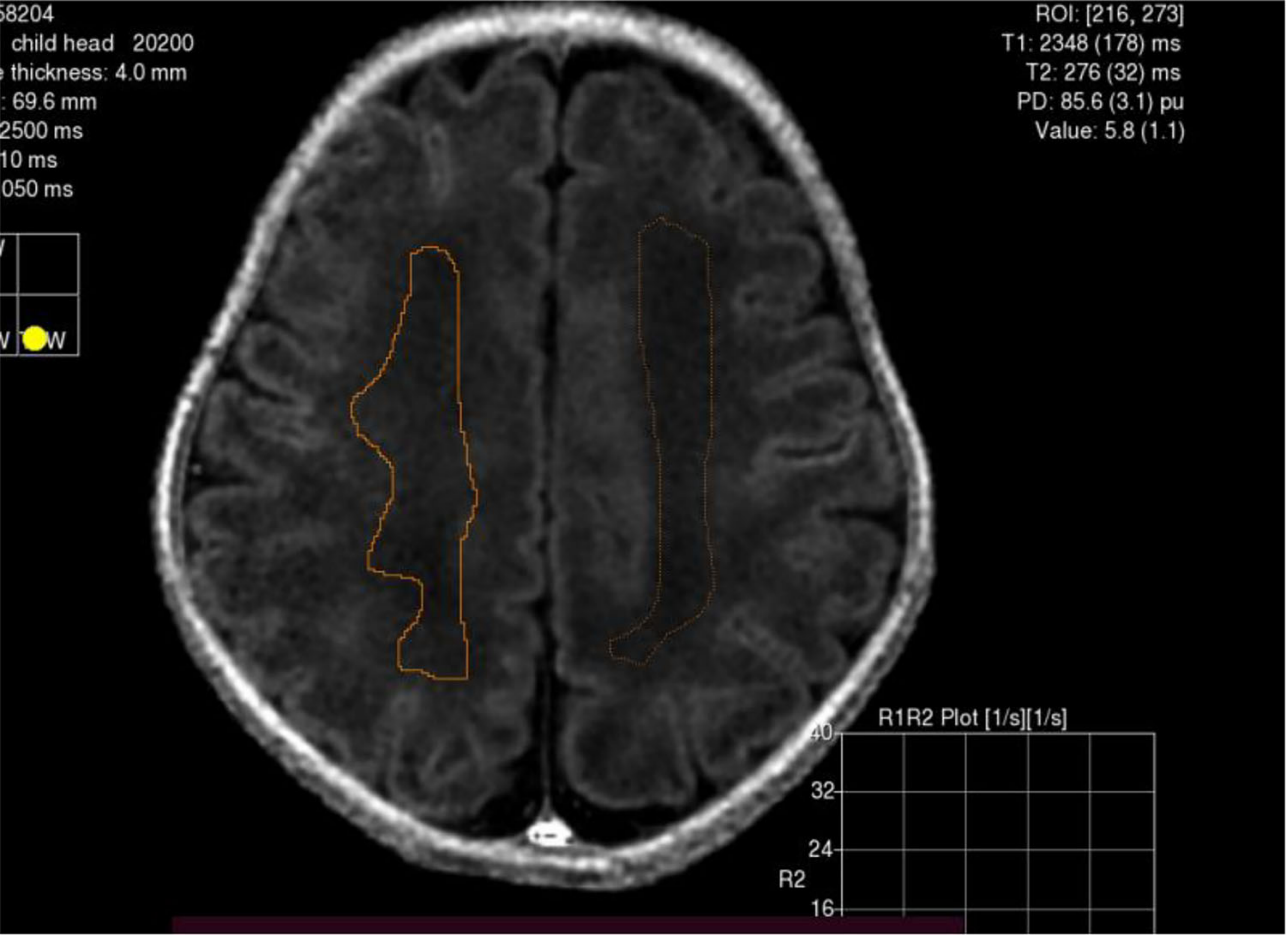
Quantitative relaxation time (T1: 2348 ± 178 ms, T2: 276 ± 32 ms) of the right centrum semiovale region of a preterm newborn were measured on MAGiC T1 FLAIR sequence.
